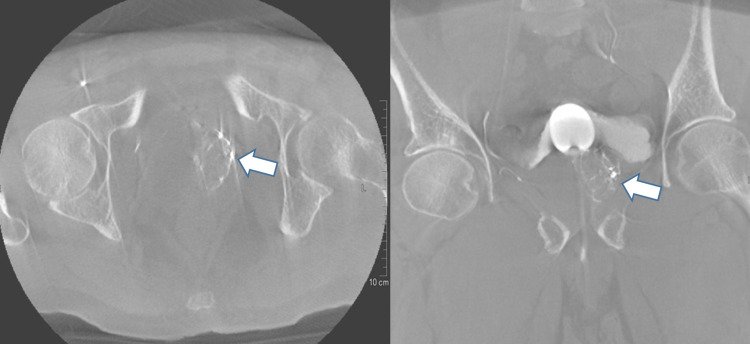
Cone beam CT (axial image, left; coronal reconstruction, right) done from microcatheter to ensure the proper position of microcatheter before embolization, showing proper opacification of the transition zone at the left side (white arrow), with no detectable opacified extra-prostatic branches confirming proper site for embolization.
